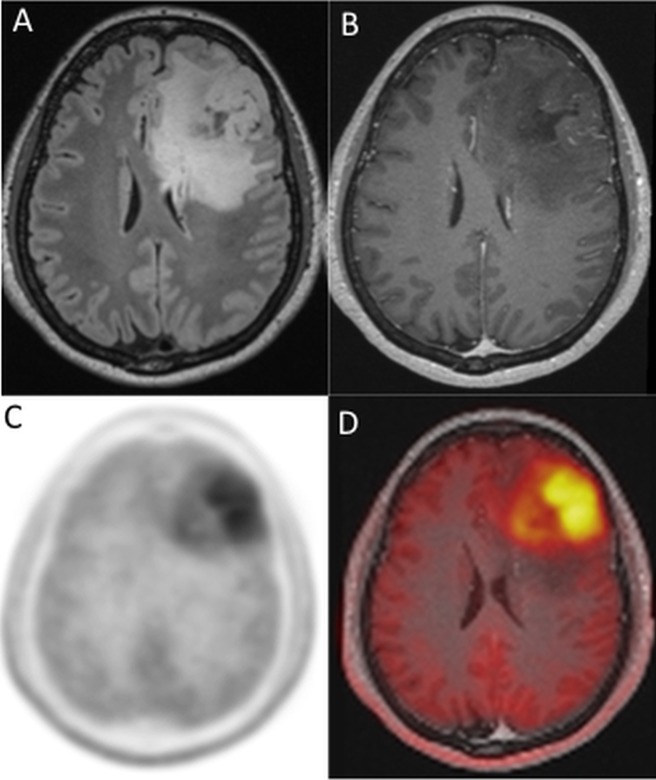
Astrocytoma WHO grade III. 20-year old woman with left frontal tumor with FLAIR- hyperintensity (A) and minimal contrast enhancement (B); leading to a diagnosis of an astrocytoma WHO grade III by MRI alone. In methionine-PET an intensive tracer more-uptake (T/N ratio 4.9) of this lesion is seen (C, D fused), making diagnosis of an astrocytoma WHO grade III with an oligodendroglial component more likely.
